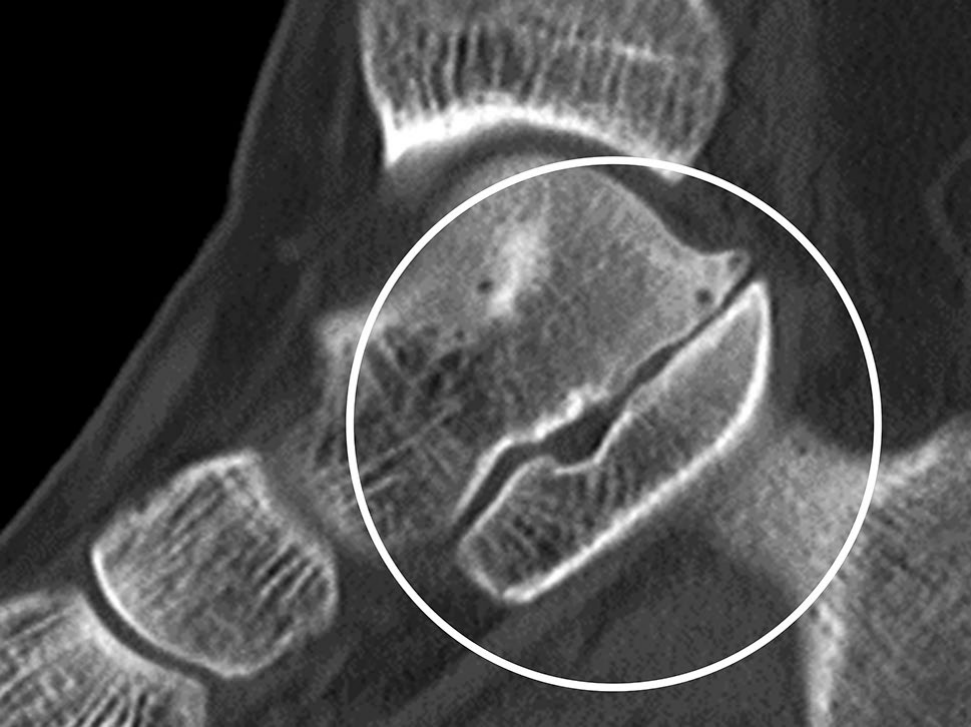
Computed tomography findings of non-osseous talocalcaneal coalition which is similar to degenerative osteoarthritis changes.
